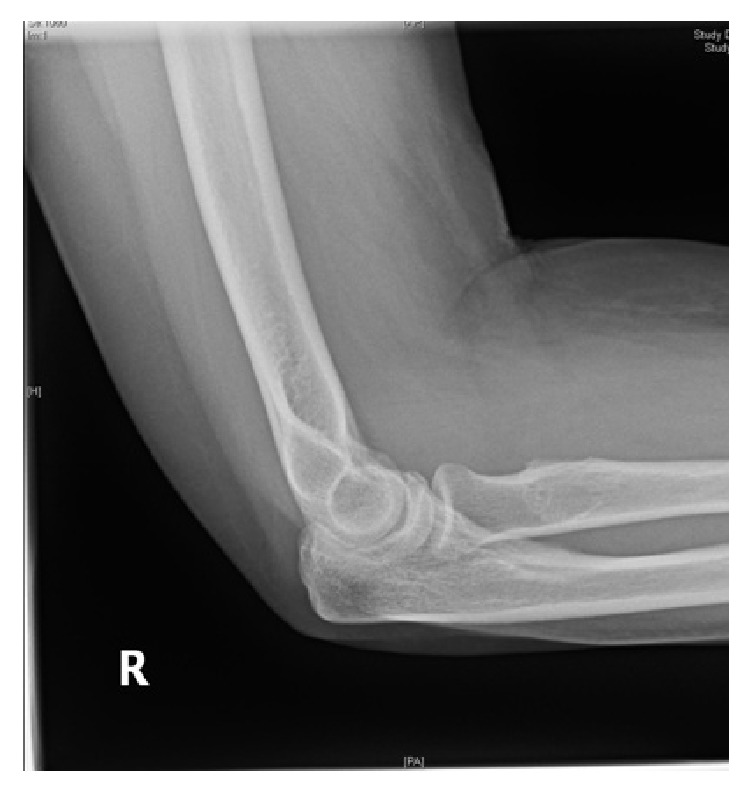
Plain radiograph at followup.
